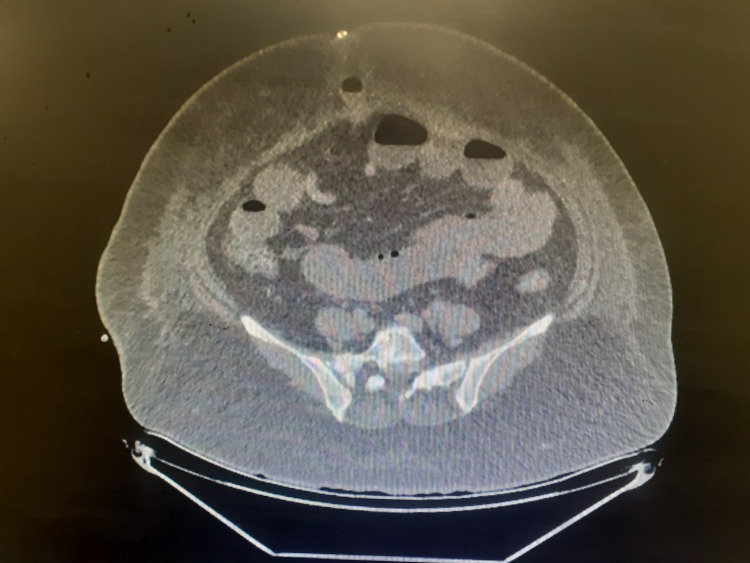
Cross-sectional cut of the CT scan at the level of the umbilicus showing the herniated small bowel loop in the umbilical port
